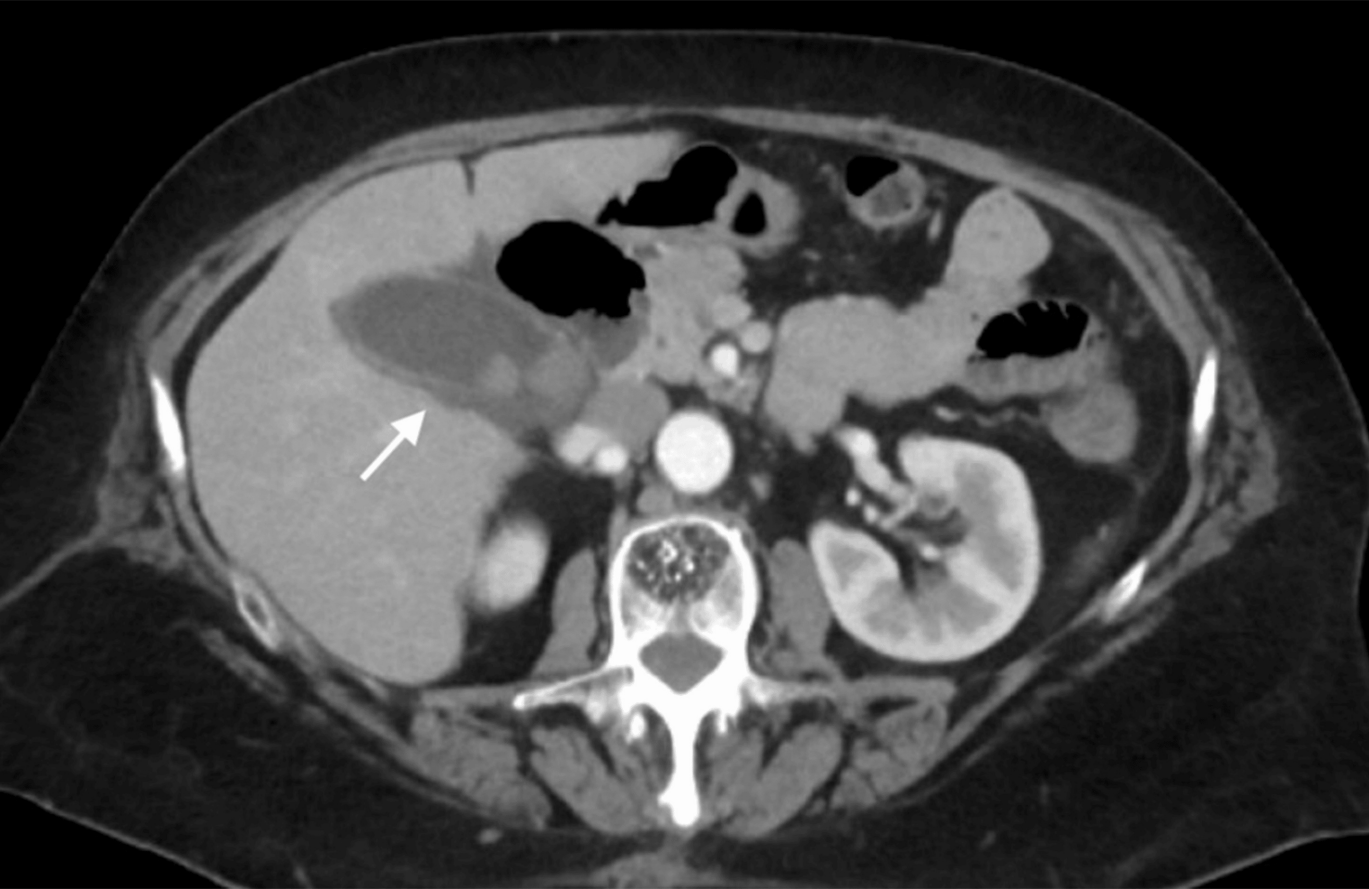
Axial CT image showing recurrent acute calculous cholecystitis The white arrow indicates a gallbladder remnant that is distended with cholelithiasis, wall thickening, and pericholecystic fluid.
